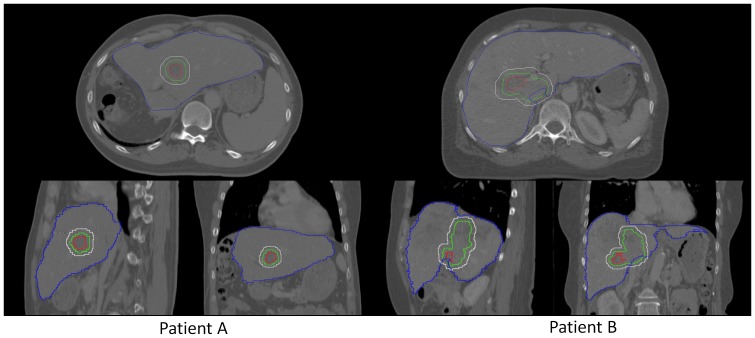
CT images and target delineations. Computed tomography for the patients with hepatocellular carcinoma included in this study. Gross tumor volumes (red), clinical target volumes (green), liver (blue), and planning target volumes for the RGPT (white) for patient A (left) and patient B (right).
